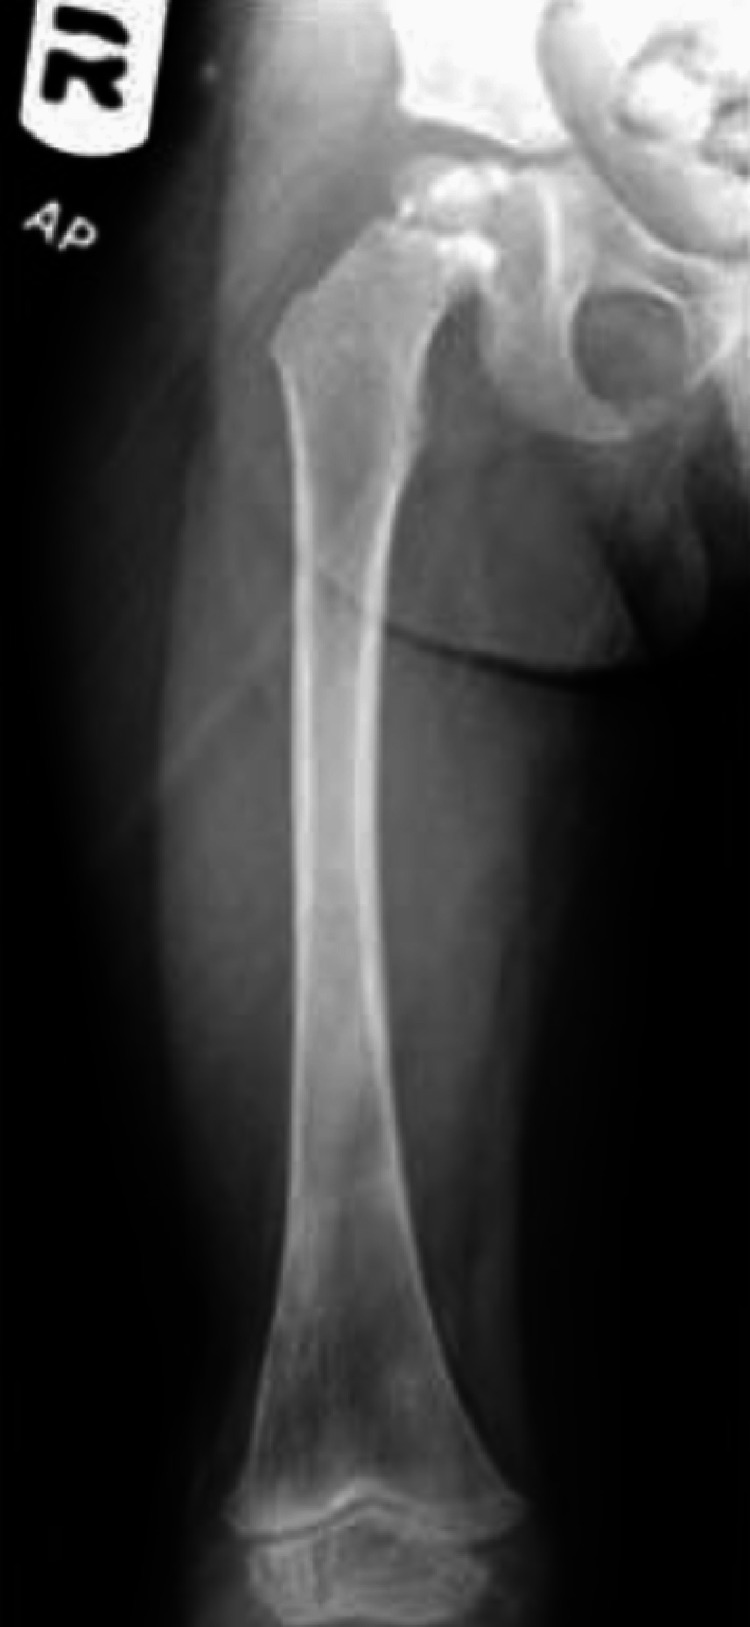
Final follow-up radiograph views showing complete resolution of the lesion
